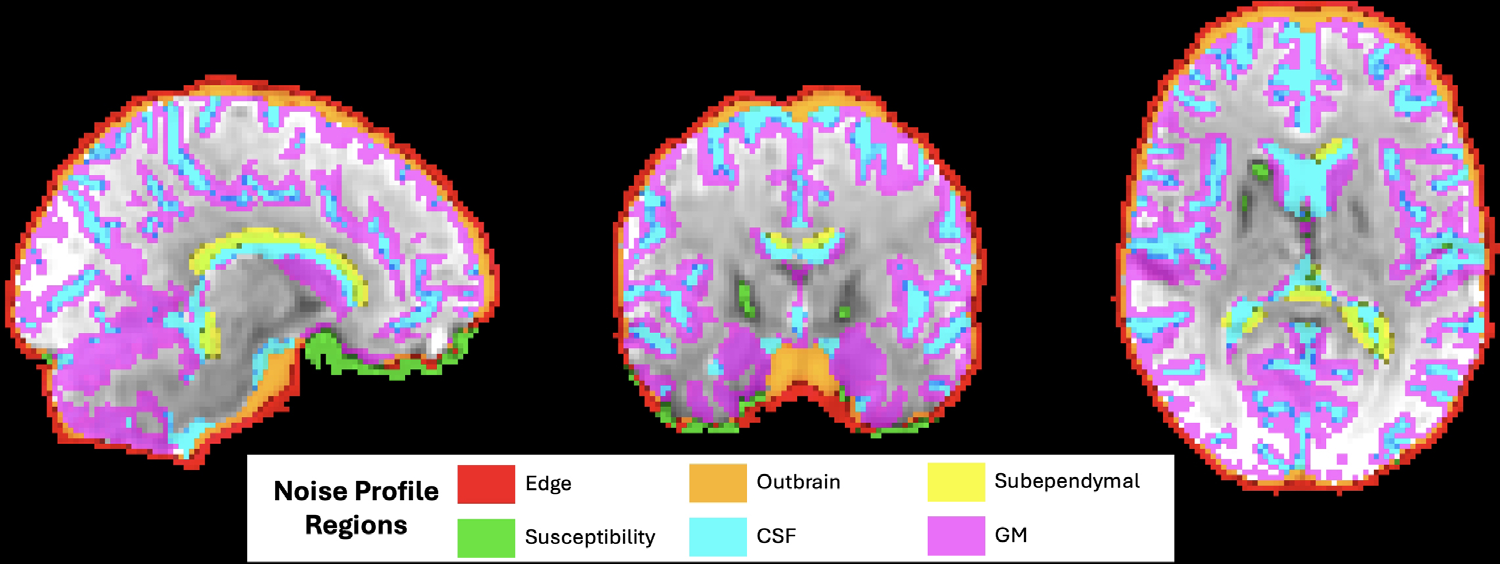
CICADA-Generated Region Masks Example. This figure displays a functional MR image with CICADA-generated region masks that help characterize commonly seen signal and noise components.
